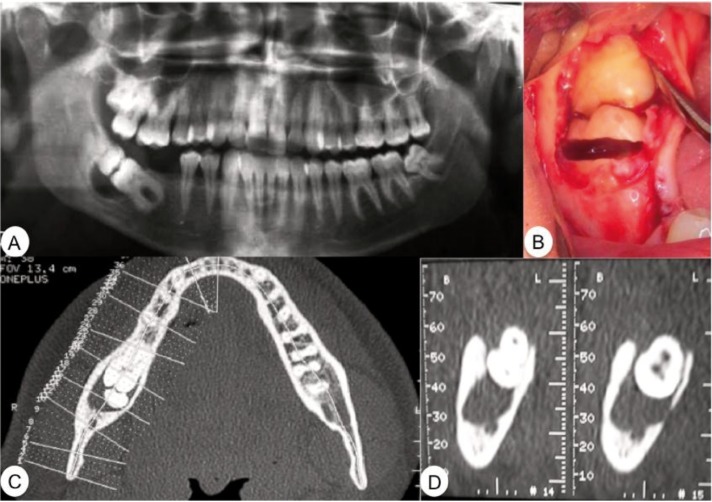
Case 1. A) Preoperative orthopantomogram. B) Cervical tooth section of the crown from tooth 37. C) Axial computed tomography. D) Computed tomography of the relationship between the molars, the dental follicle, and the mandibular canal.
